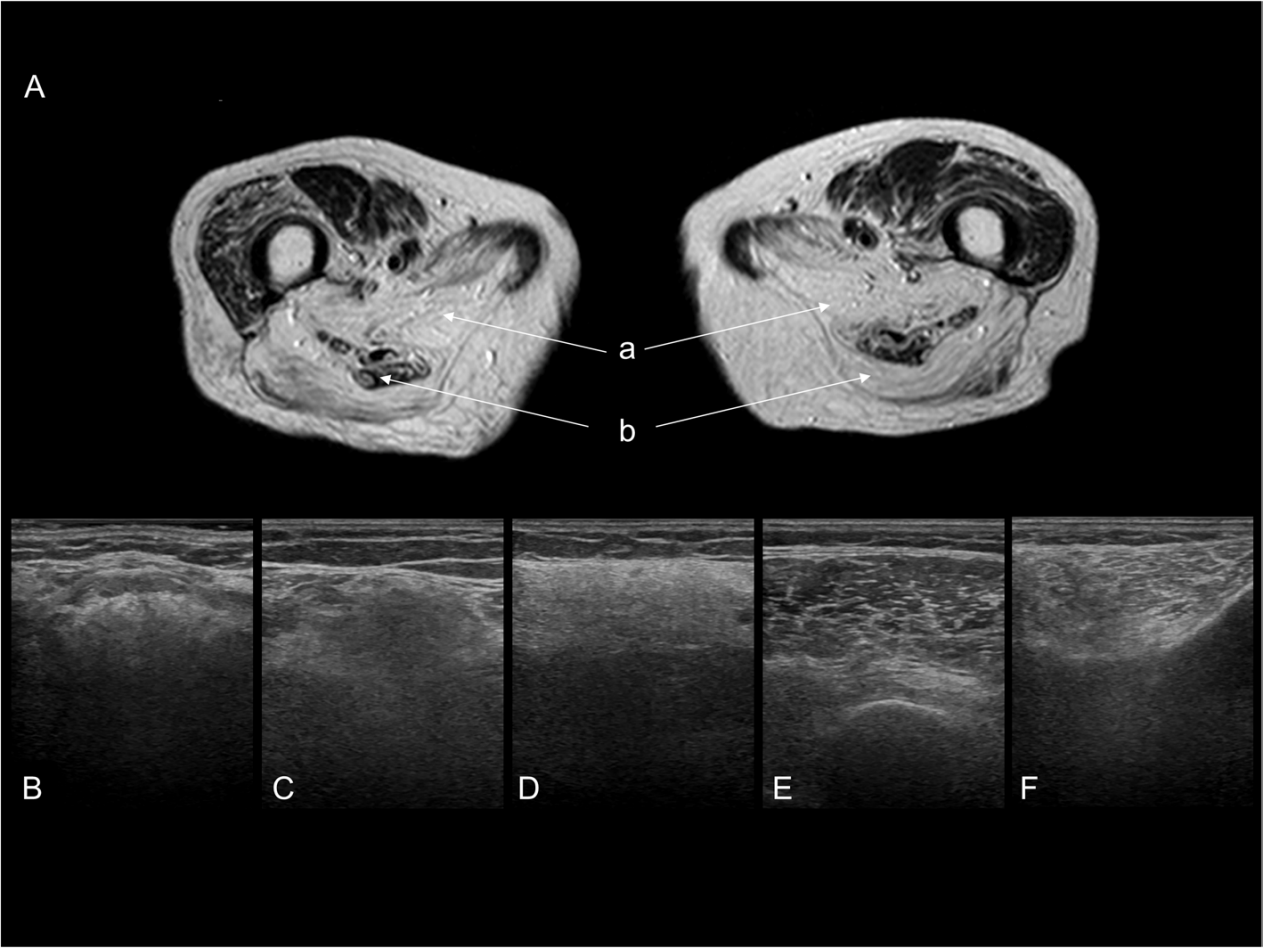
Axial T2-weighted muscle MRI at the thigh level (A) and muscle ultrasound images (B-F). On MRI, muscles in the posterior compartment (biceps longus head (a) and adductor magnus (b) muscles) showed a high signal intensity, whereas muscles in the anterior compartment were preserved (A). Muscle ultrasound also showed a posterior dominant-involvement pattern in lower limb muscles in addition to tongue muscle involvement. The tongue (B), biceps femoris (C), and gastrocnemius (D) muscles had a diffusely hyperechoic pattern, whereas normal muscle structures were relatively preserved in the vastus lateralis (E) and tibialis anterior (F) muscles
